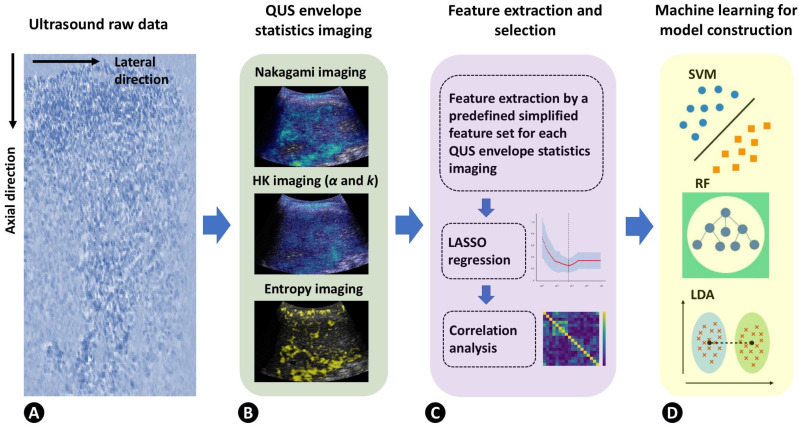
The algorithm of ultrasound scatteromics analysis, including (A) using ultrasound raw data as the input; (B) QUS envelope statistics imaging is performed using raw data; (C) feature extraction by a predefined simplified feature set, and feature selection is performed by LASSO regression and correlation analysis; (D) model construction by machine learning.
